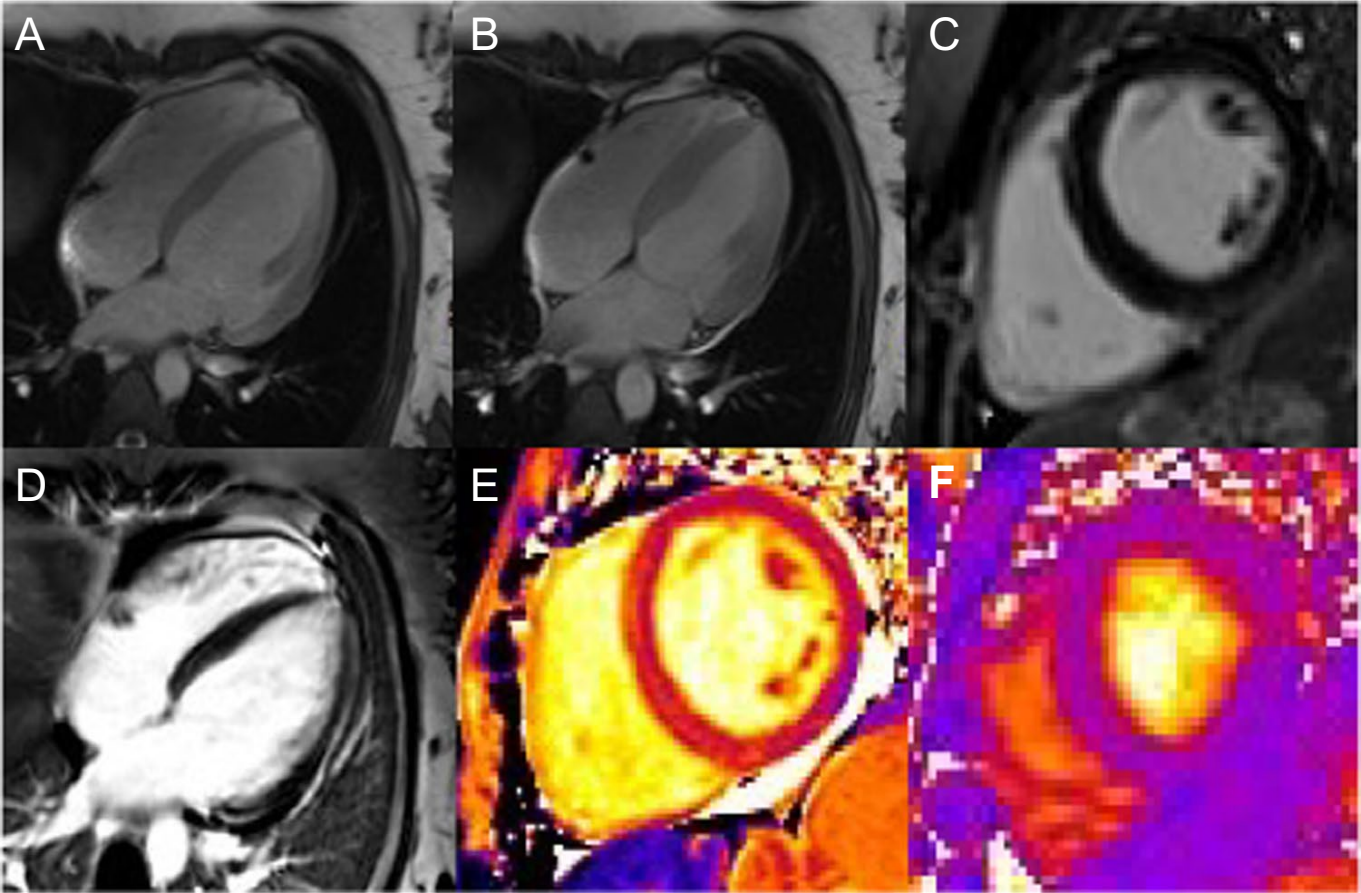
Non-sustained ventricular tachyarrhythmia and dyspnea in a 31-year-old woman. A 31-year-old primiparous woman with a history of polycystic ovary syndrome and obesity (body mass index 33 kg/m^2^) and no family history of CVD was referred to hospital for a planned caesarian section. Her pregnancy has been complicated by hypertension and gestational diabetes. The aftermath of delivery was marked by episodes of non-sustained ventricular tachyarrhythmia and dyspnea. Troponin and NT-proBNP were within normal range. An echocardiography revealed a mildly reduced LVEF (40%) without regional wall motion abnormalities and relevant valvular abnormality, as well as normal right-sided cardiac cavities. CMR was performed confirming a mildly reduced LVEF of 42% (panels A and B) with no regional component, particularly no pattern suggestive of TTC. LV and RV were non-dilated (respectively, LV end-diastolic volume 85 mL/m^2^, N: 66–101 mL/m^2^; and RV-end-diastolic volume 80 mL/m^2^, N: 65–111 mL/m^2^), hence excluding preexisting cardiomyopathies and DCM. No myocardial LGE was detected (C), excluding necrosis, and pharmacological stress revealed no ischemia (D). T1 (E) and T2 (F) mapping of the LV myocardium were normal, 1247 ms (N: 1222 ± 42 ms) and 39 ms (N: 38 ± 2.3 ms), respectively, thus excluding scar and edema/myocarditis. Based on the timing of the disease (early postpartum) and other cardiomyopathies being excluded, the diagnosis of PPCM was made. Dyspnea during or in the direct aftermath of pregnancy is a common situation, which can result either from hemodynamic adaptations to pregnancy or from complications. The differential diagnosis in this setting includes pulmonary embolism and PPCM. Key to the diagnosis of PPCM is the timing of the disease and the absence of previous cardiomyopathies. Advanced noninvasive imaging is helpful in this setting, to establish LV systolic dysfunction and to rule out concurrent cardiomyopathies. Abbreviations: *CVD*: cardiovascular disease; *CMR*: cardiac magnetic resonance; *DCM*: dilated cardiomyopathy; *LGE*: late gadolinium enhancement; *LV*: left ventricle; *LVEF*: left ventricular ejection fraction; *N*: normal; *NT-proBNP*: N-terminal brain natriuretic peptide; *PPCM*: peripartum cardiomyopathy; *RV*: right ventricle; *TTC*: Takotsubo cardiomyopathy
